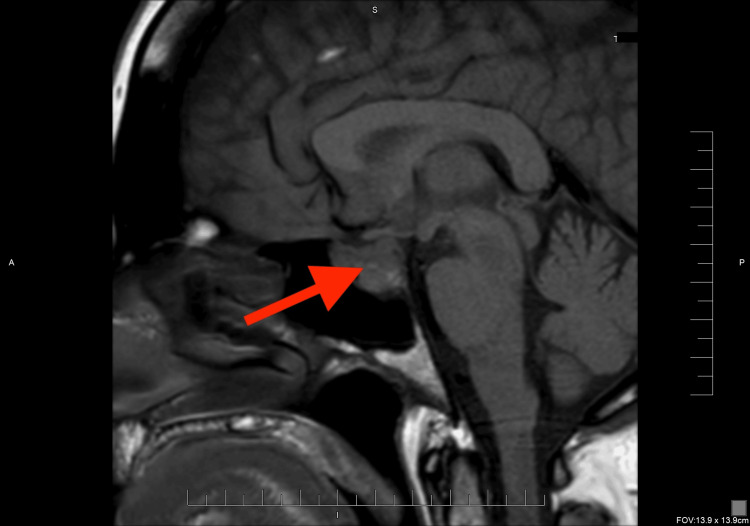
Sagittal non-contrast T1-weighted MRI Midline T1 sequence demonstrates an iso-to-hypointense 2.3 × 1.5 × 1.2 cm sellar mass (red arrow) that elevates the diaphragma sellae and indents the optic chiasm while extending into the left cavernous sinus.
